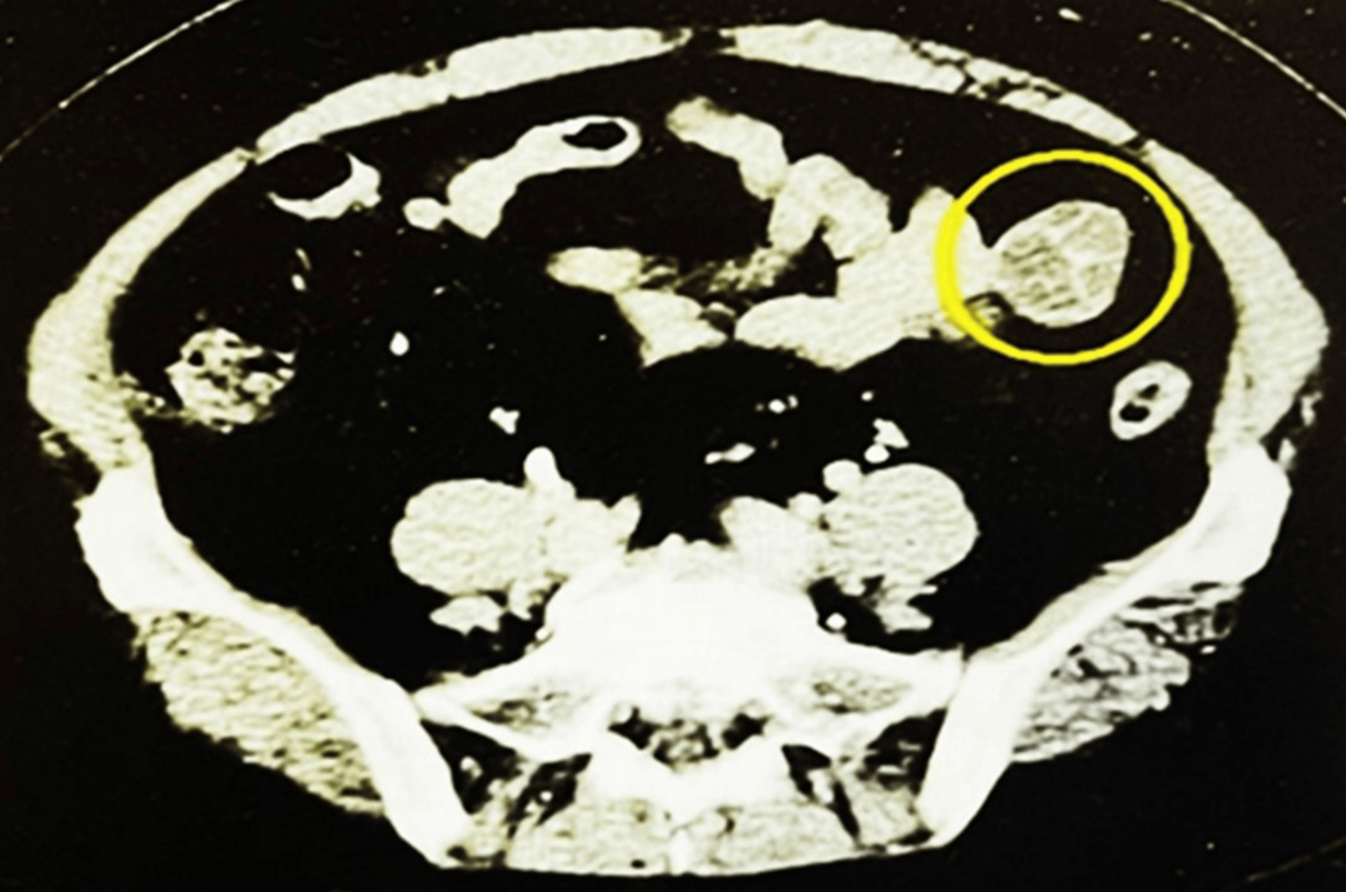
Abdominal computed tomography A protruding tumor is observed adjacent to the intestinal wall (circled). The intratumoral space is separated by septa containing low-density contents.
